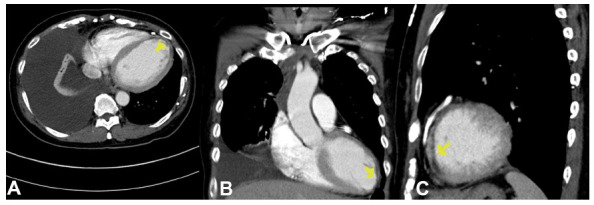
Left Ventricular Myocardial Infarction Detected on Routine Non-Gated Thoracic CT.
